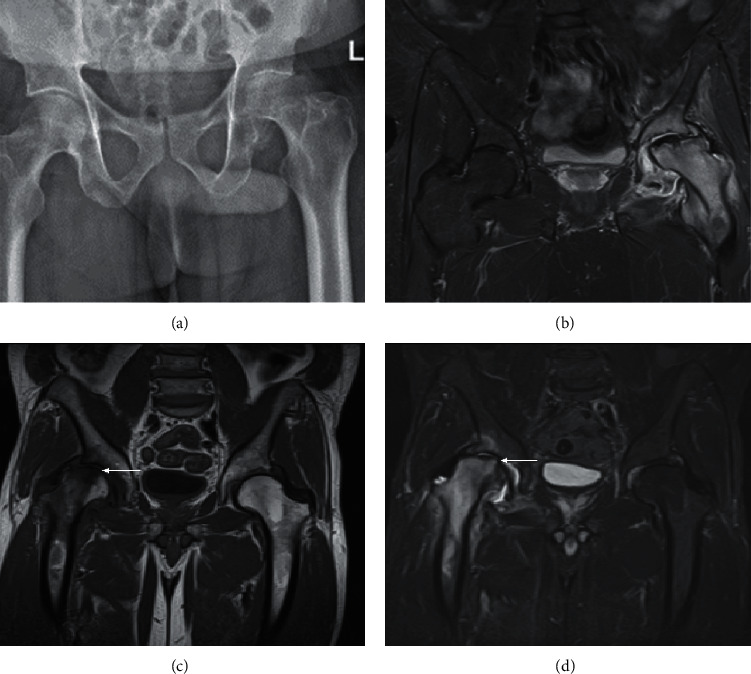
Two cases of rapidly progressive hip osteoarthritis. X-ray (a) and coronal STIR (b) of a 78-year-old man presenting with an increasingly painful and stiff left hip, demonstrating left hip joint effusion, joint marrow oedema signal, and flattening of the femoral head. Joint aspirate and subsequent washout were negative for infection. Coronal T1 (c) of a second patient with coronal STIR (d) performed 2 months apart shows the rapidly progressive nature of this condition, with hatchet-like deformity of the femoral head in the latter study (d arrow) due to subchondral insufficiency fracture.
